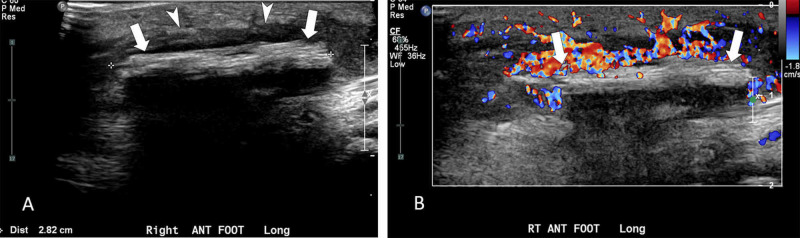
Longitudinal greyscale and color Doppler of the right foot Longitudinal grayscale (A) and color Doppler (B) ultrasound images obtained on re-admission show an echogenic structure (arrows), believed to represent a foreign body measuring approximately 2.8 cm in length and located approximately 10 mm deep to the skin surface. Hypoechoic granulation tissue (arrowheads in A) is seen adjacent to the foreign body, which is hypervascular on color Doppler interrogation (B).
